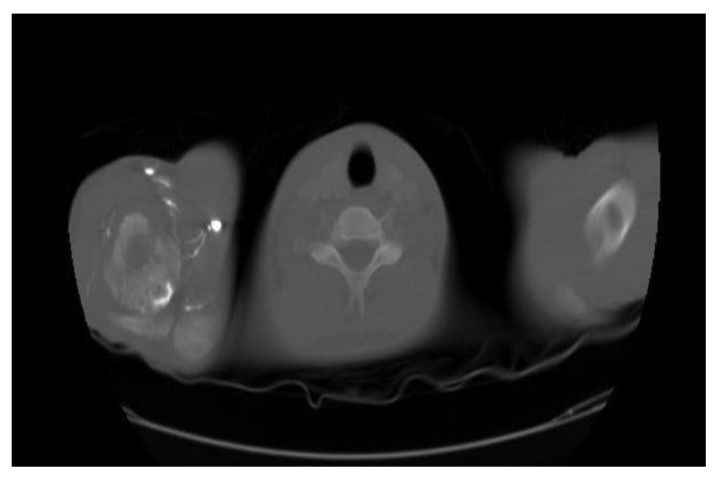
CT both shoulder axial view (bone window) showing irregular destructive lytic lesions of right upper humerus.
